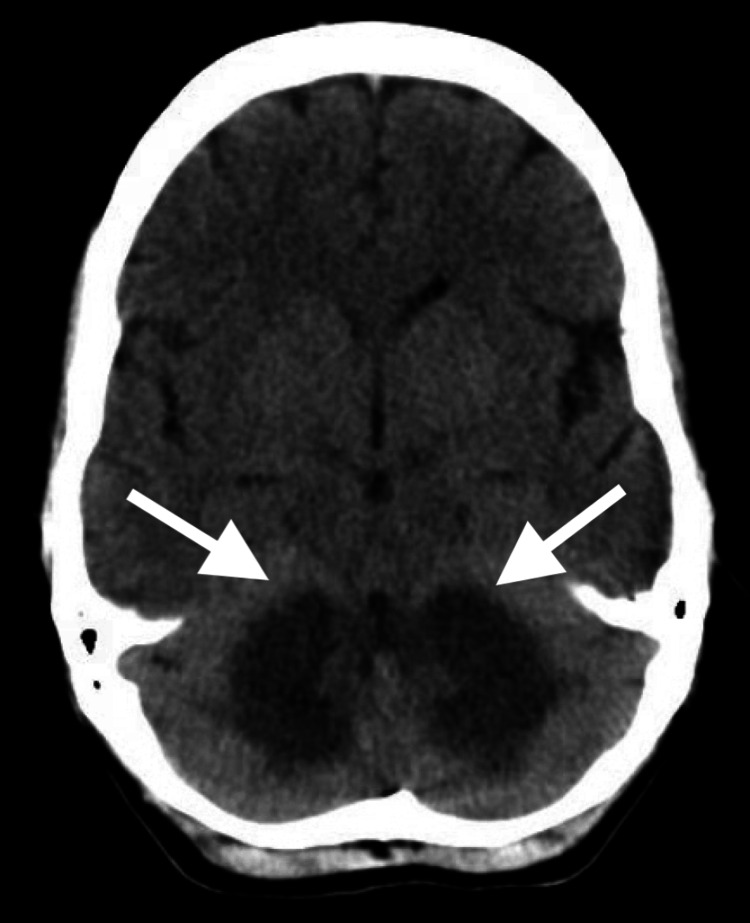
Noncontrast CT scan of the brain demonstrating diffuse hypoattenuation in the cerebellar white matter (arrows).
